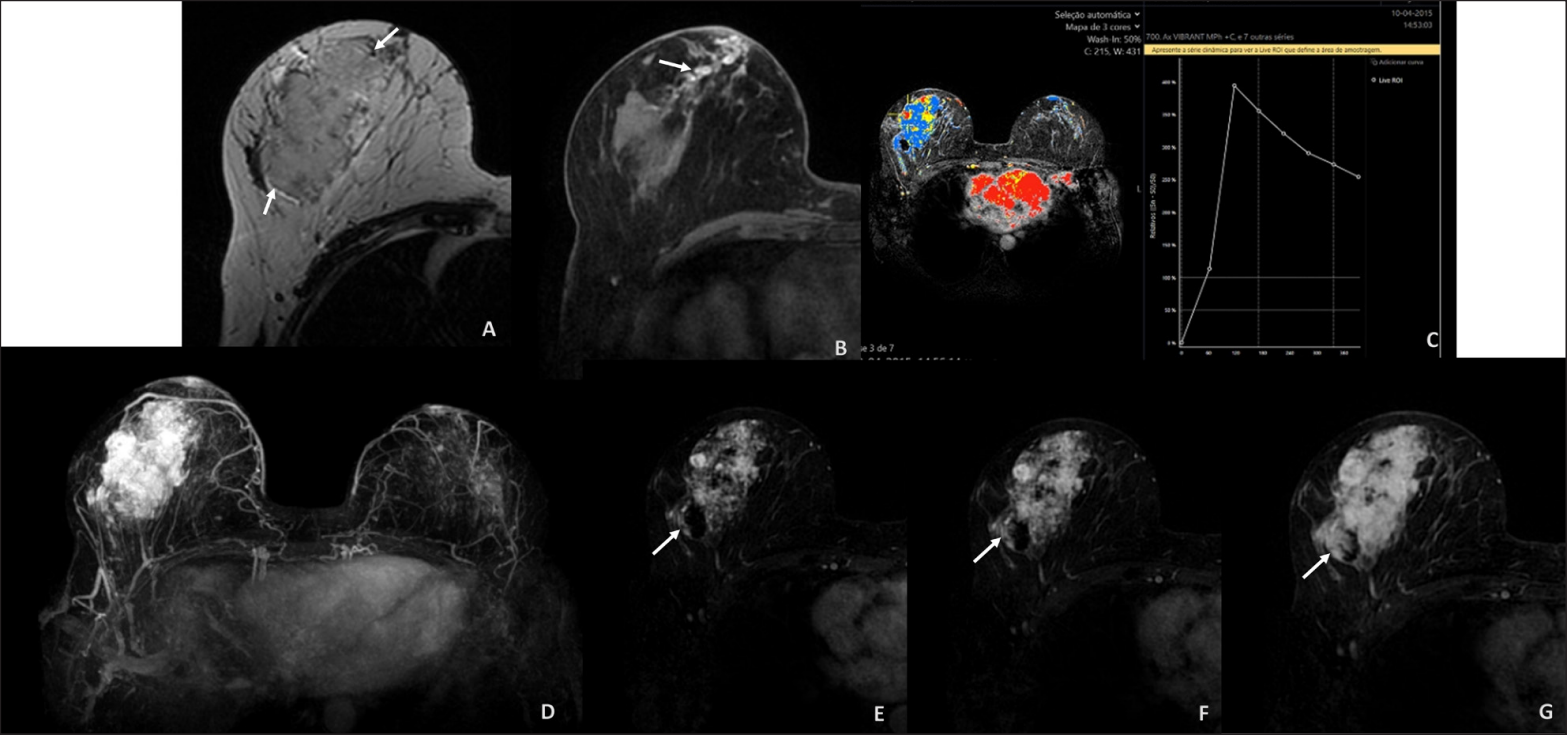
Ultrasound-guided core biopsy confirmed the diagnosis of primary breast angiosarcoma (PBA). The patient underwent right total mastectomy and adjuvant radiotherapy.
